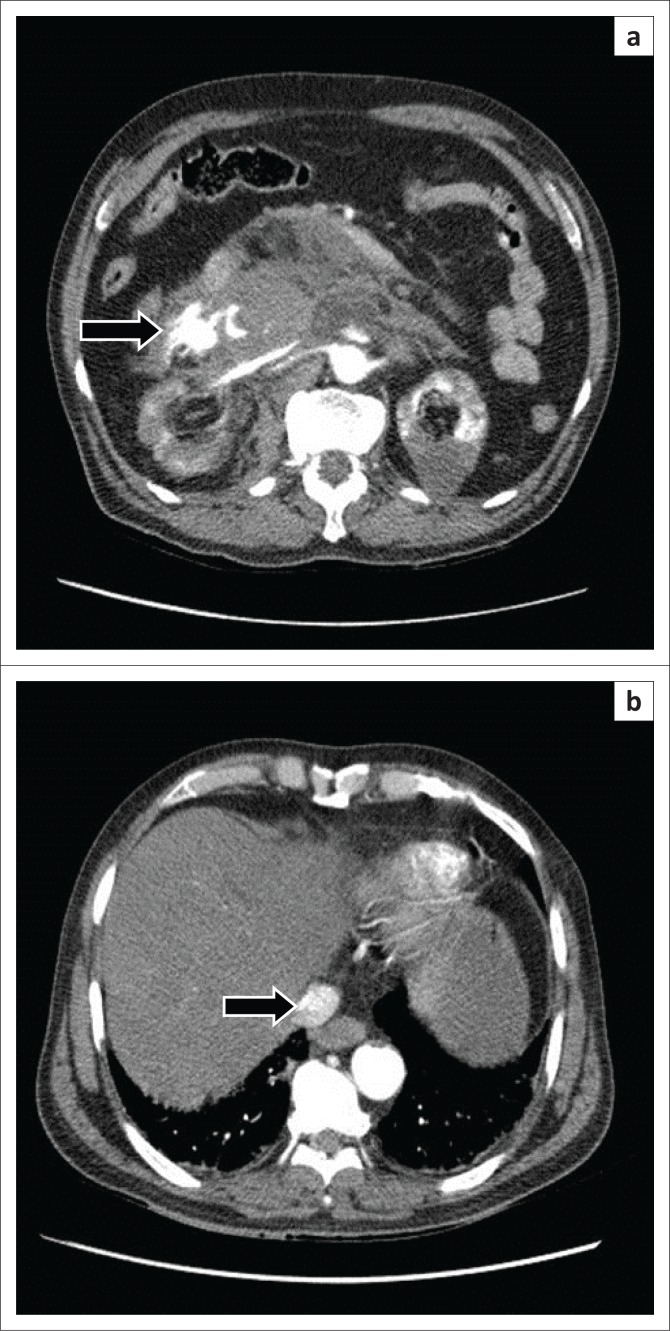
(a) Axial contrast-enhanced computed tomography scan demonstrating active extravasation of contrast into the peritoneal cavity (arrow). Contrast is also seen in the renal veins; (b) Contrast seen in the hepatic inferior vena cava (arrow) but with less attenuation (HU – 150) owing to reflux from right atrium.
